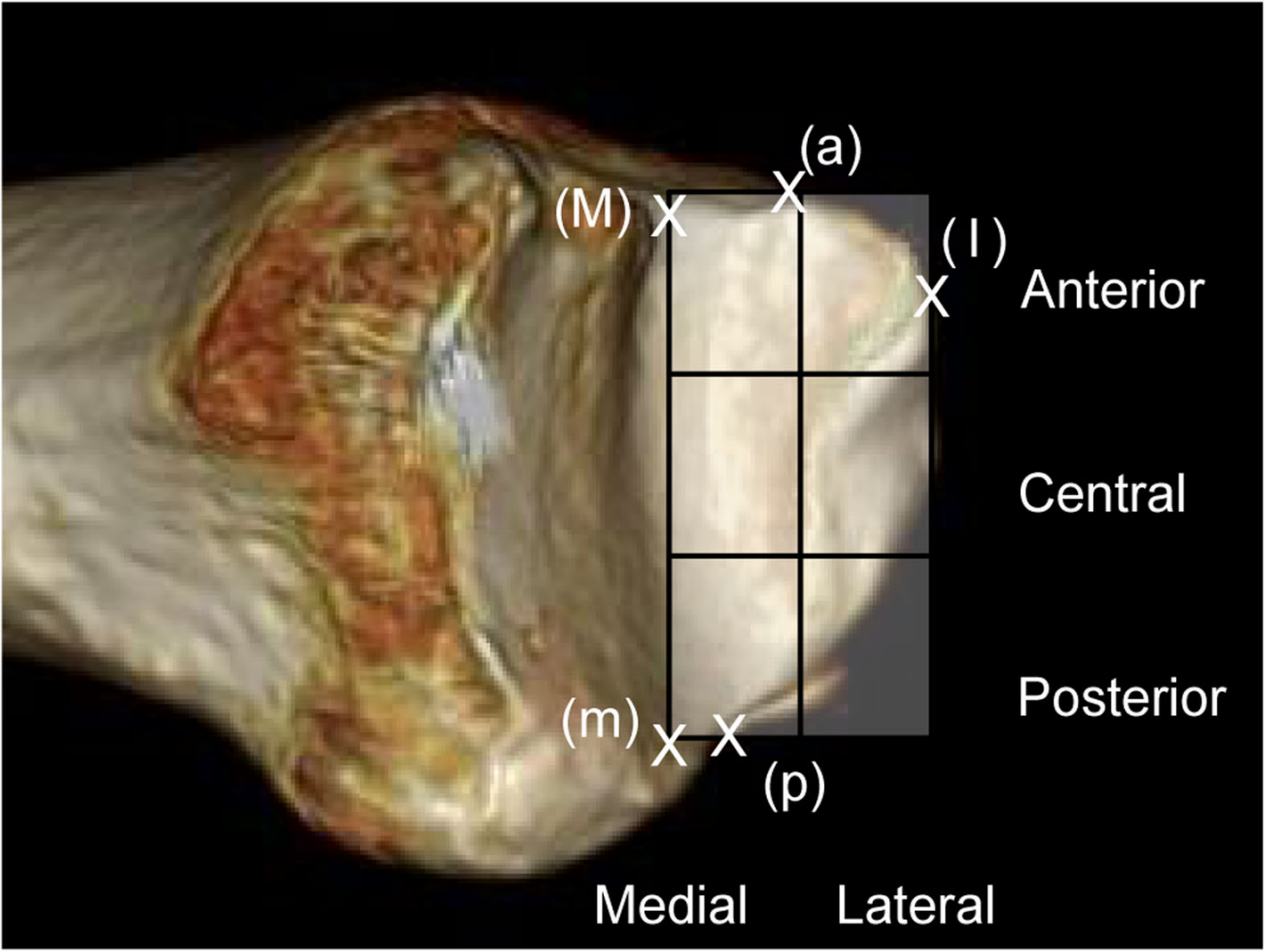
Malleolar surface image of the tibia with 3D CT. Assessment was performed using the line Mm, anterior border (a), posterior border (p), and distal portion (lateral border) (l). The rectangle was divided into three sections (anterior, central, and posterior) anteroposteriorly and two sections mediolaterally
